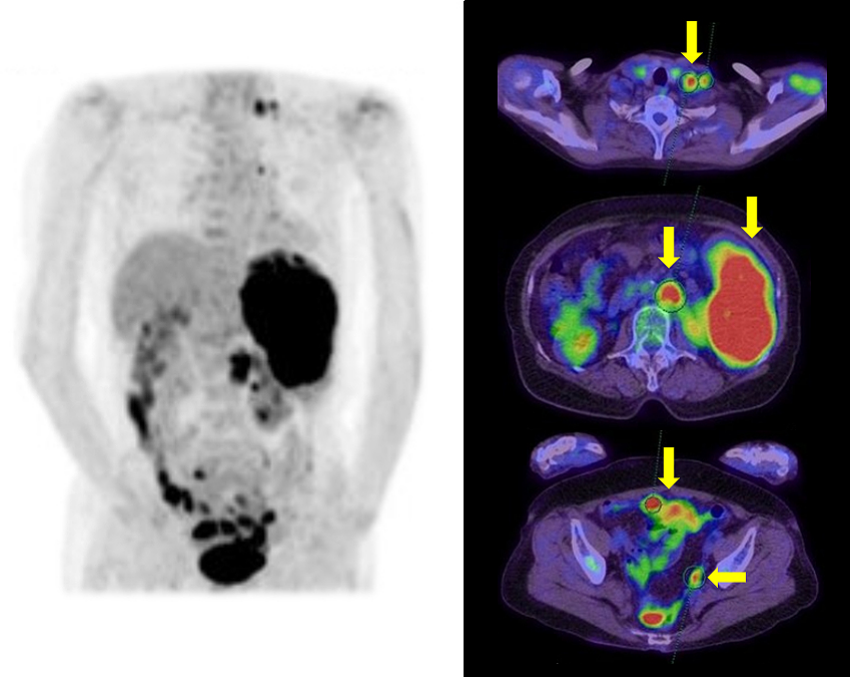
Positron emission tomography (PET)/computed tomography (CT) showed abnormal accumulation in the spleen, the para-aortic and Virchow’s lymph node, the intestine, and the pouch of Douglas (arrows).
